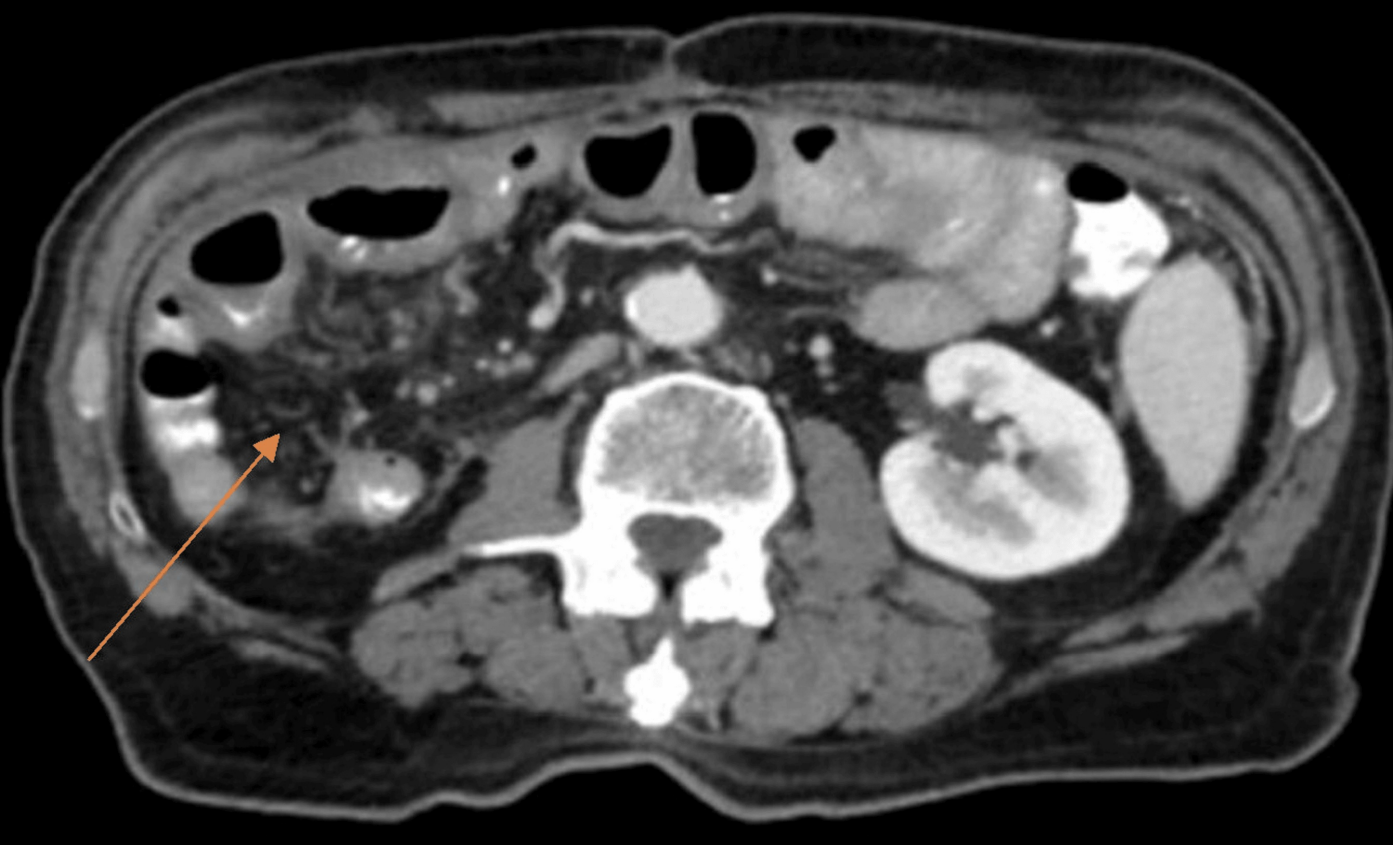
Axial computed tomography scan showing the absence of a solid tumor lesion, previously described (arrow)
